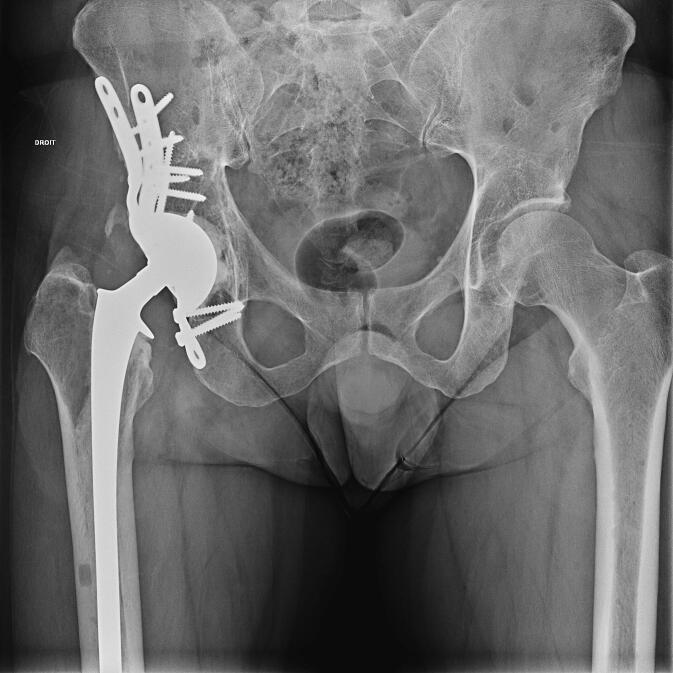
A pre-operative radiograph of a 46-year-old man with a multiple myeloma involving the acetabulum. B: pre-operative CT scan. C: Post-operative radiograph. The patient had a total hip arthroplasty with a partial pelvis implant.
